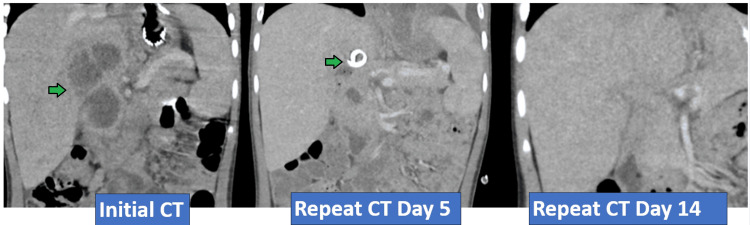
CT depiction of complex septated fluid collection in the hepatic hilum before and after intervention. Green arrow in initial CT and repeat CT day 5 indicates complex septated fluid collection in the hepatic hilum.
